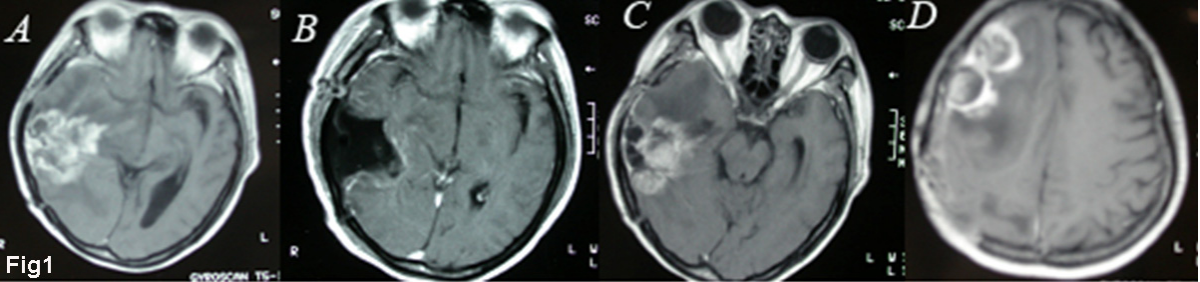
MRI images of a 52-year-old female patient who underwent two operations with an interval of 6 months because of the rapid relapse of her tumor. A, The primary tumor located in the right temporal lobe and pathologically diagnosed as mixed glioma composed of anaplastic ependymoma and astrocytoma (WHO grade III). B, Post-operation image showing nearly total resection of the tumor. C, D, Relapse occurring 6 months later in situ and the ipsilateral frontal lobe; the recurrent lesion had transformed and progressed into glioblastoma multiforme (WHO grade IV).
